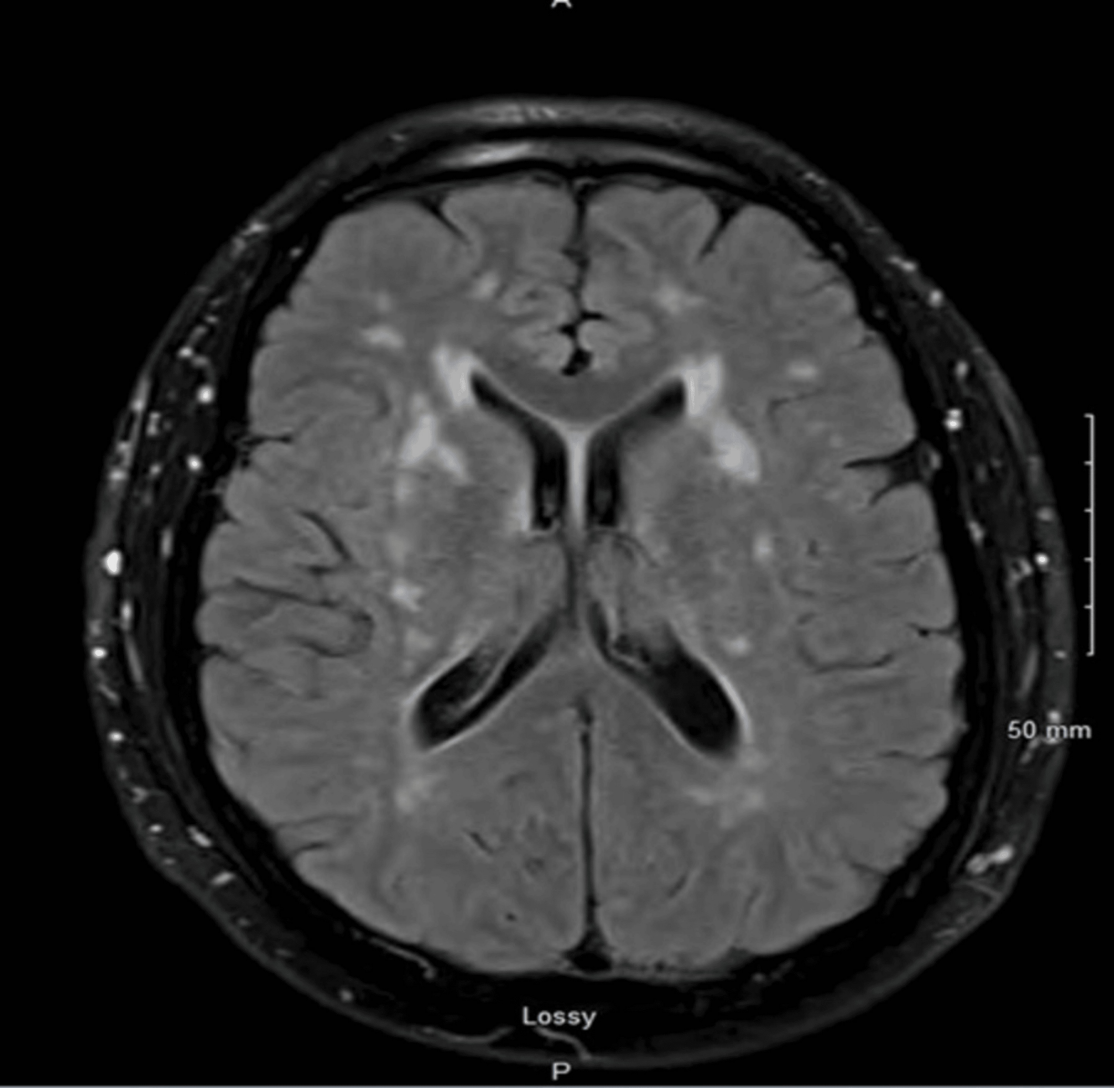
MRI images showing multiple prior infarcts, especially around the basal ganglia
